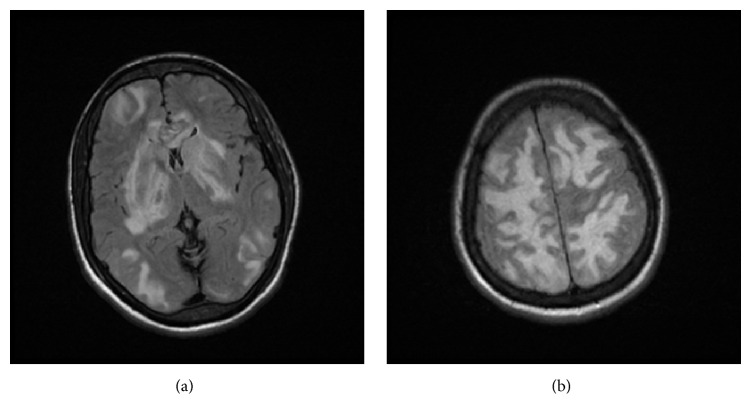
MRI Brain-Axial FLAIR-weighted images demonstrate the multifocal areas of edema involving the subcortical and deep white matter as well as the deep grey structures. Edema is also noted in the genu of the corpus collosum.
